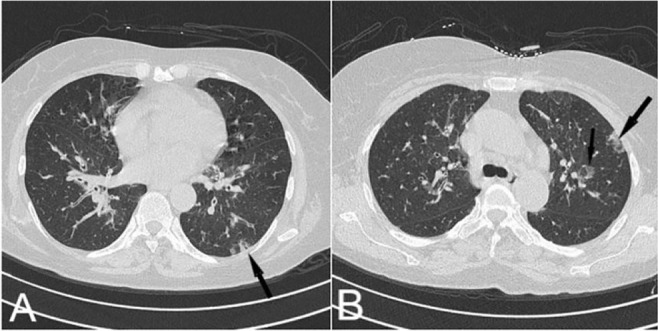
A,B)Non-contrast axial chest CT scan of 42-year-old woman, patchy Peri-bronchovascular GGO and thickening (arrows) is seen during the epidemic of H1N1, compatible with influenza pneumonia.
